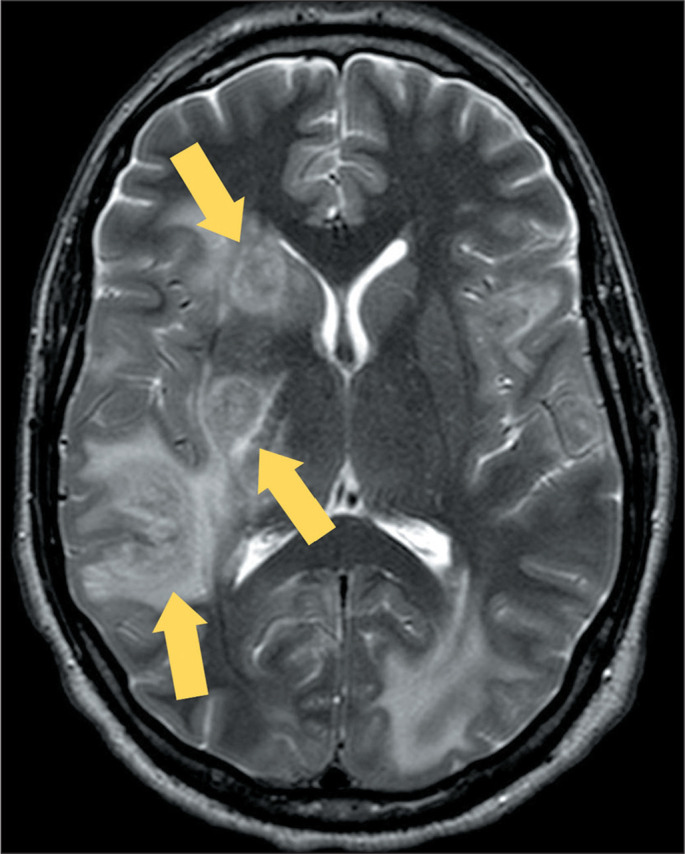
Cerebral aspergillosis. MRI of a 40-year-old patient with acquired
